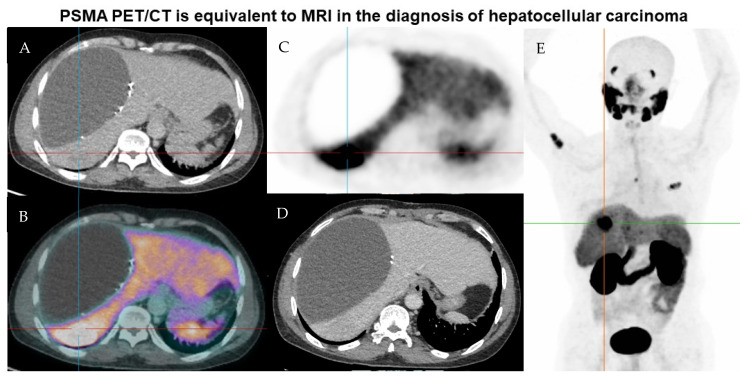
Representative imaging of a lesion suspicious for recurrence and metastatic spread of HCC on PSMA PET. Case details: 58-year-old male of Asian ethnicity with previous resection of HCC in 2019. (A) low dose CT, (B) fused PET PSMA and low does CT, (C) PSMA PET, (D) contrast-enhanced CT, (E) MIP PSMA PET image. There is a hypodense fluid attenuation area within the right liver adjacent to surgical clips representing a known seroma/biloma. Ultrasound guided biopsy of the PSMA avid lesion in hepatic segment 7 confirmed HCC. The patient was commenced on lenvatanib therapy with PSMA avid-likely bony metastatic disease in the right proximal humerus, T5 vertebral body and left 3rd rib anterolaterally. The liver lesion was non-enhancing on contrast-enhanced CT.
